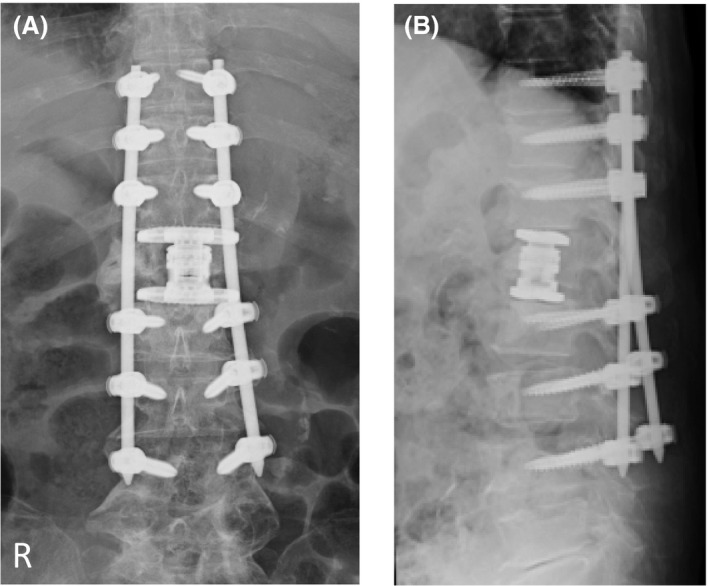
Plain postoperative radiographs. Results of postoperative examination with plain radiography: (A) anteroposterior view and (B) lateral view. The alignment of the posterior wall of the vertebrae prevented projection into the spinal canal. Rectangular endcaps were fitted between the inferior T12 and superior L2 endplate.
